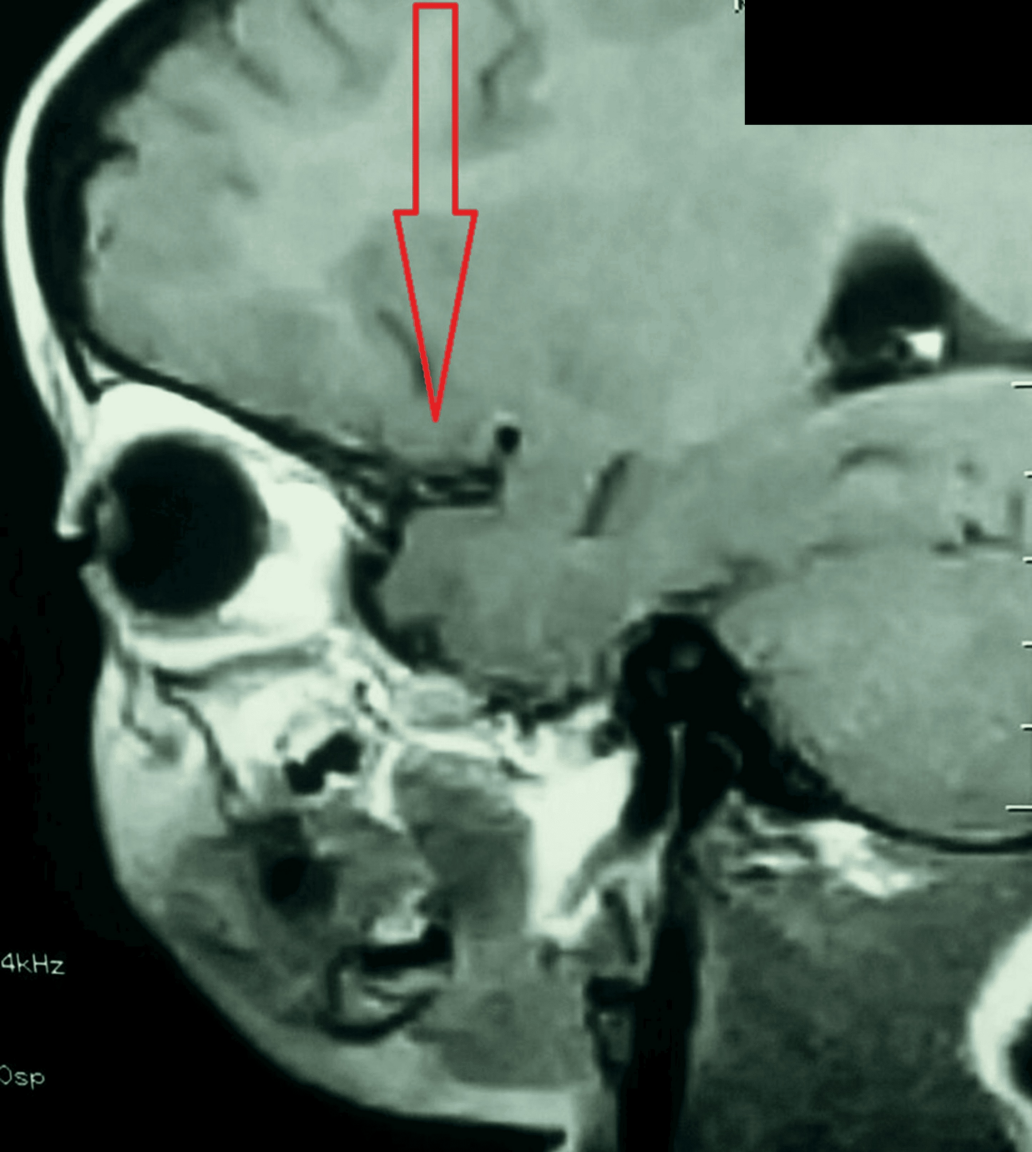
MRI demonstrated an intracranial component of the lesion located anterior to the sella turcica, abutting the inferior surfaces of the bilateral frontal lobes. The lesion (red arrow) extended inferiorly through the anterior skull base into the submucosal region of the roof of the oral cavity. MRI: magnetic resonance imaging
